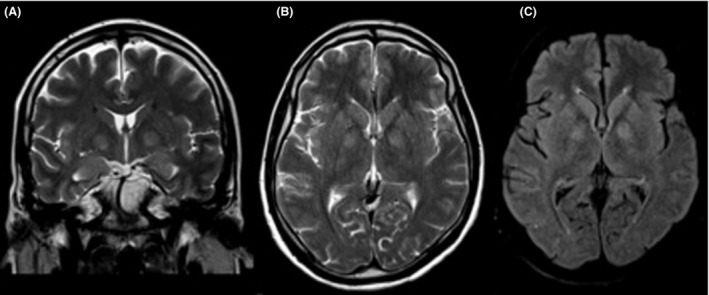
Patient's brain MRI reveals signal abnormalities in basal ganglia, with no mass effect in (A) coronal T2 TSE, (B) axial T2 TSE, and (C) axial T2 FLAIR.
